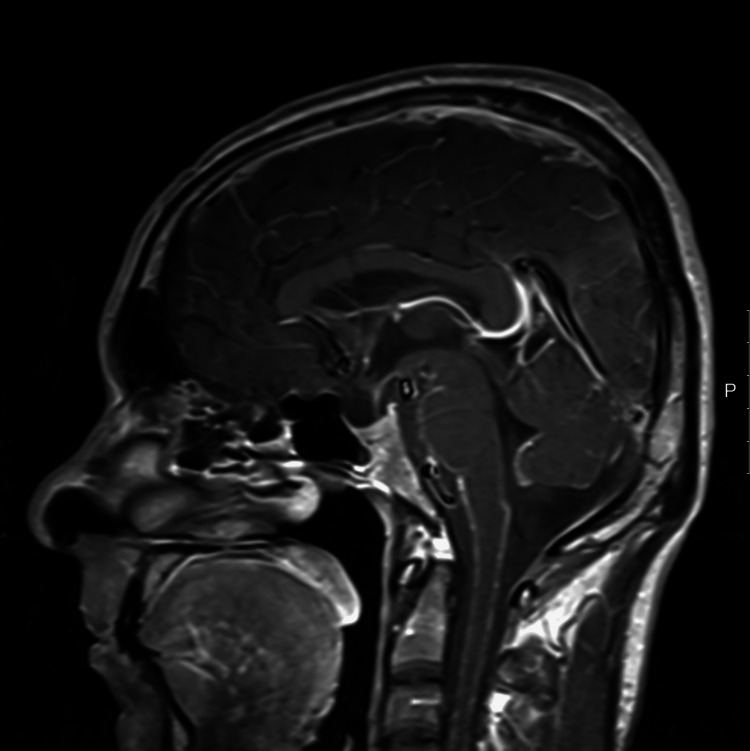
MRI brain scan with contrast showing perimesencephalic and cervical spinal cord leptomeningeal enhancement, as well as cranial nerve enhancement
